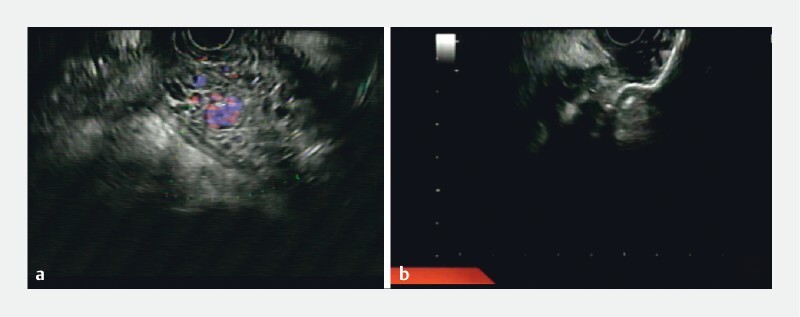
Endoscopic ultrasound view of the walled-off pancreatic necrosis (WOPN).
